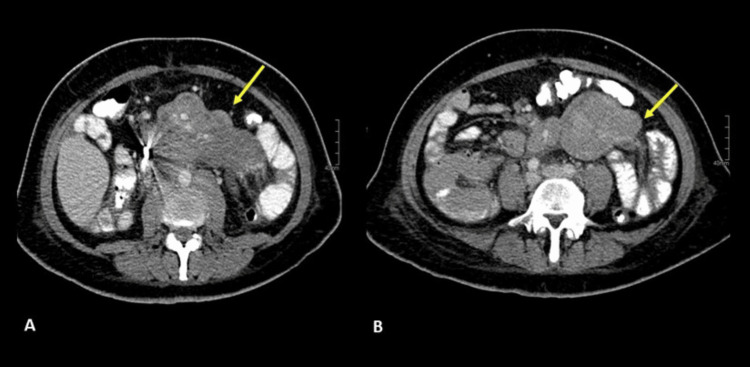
Contrast-enhanced abdominal CT scan showing confluent mesenteric, peri-celiac, and retroperitoneal lymphadenopathy (yellow arrows in A and B) surrounding the mesenteric vessels without invading them, with some central hypodense areas due to necrosis.
